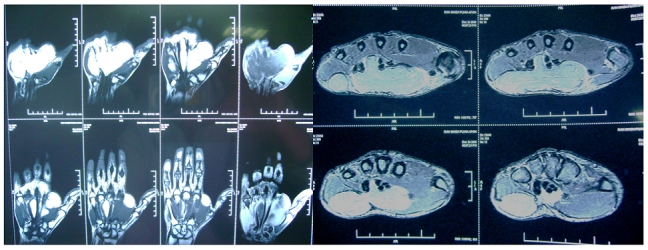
MRI showing actual extent of tumor (bright signal on T1 images -transverse and coronal sections)
